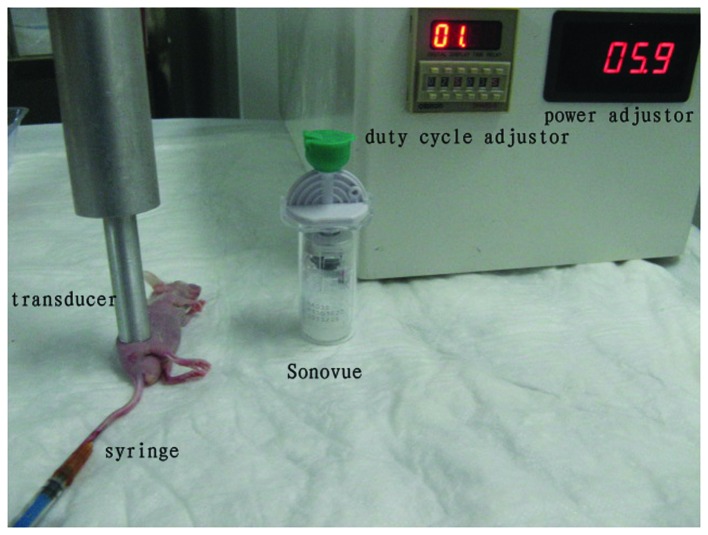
Therapy apparatus for low-frequency US and experimental set-up. US, ultrasound.
